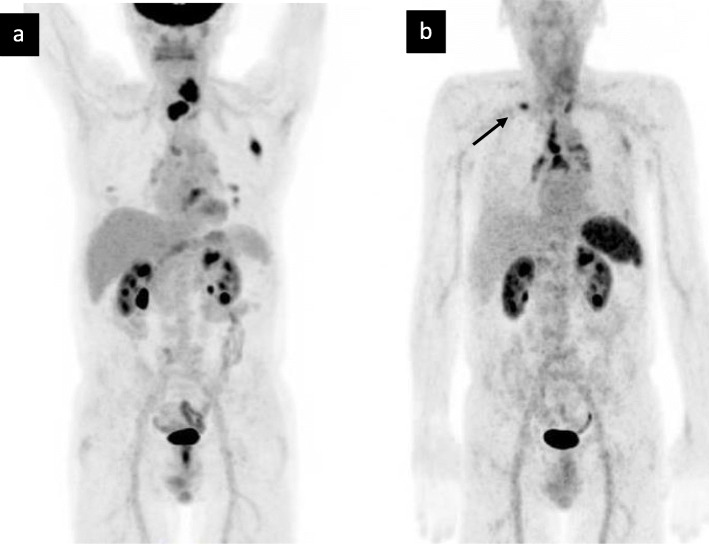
Key lesion rather peripher located to the primary tumor. Disconcordance of (a) FDG PET/CT and (b) non-invasive Pentixafor PET/CT imaging in one patient suffering from esophageal cancer. The lesion demonstrates high CXCR4 expression; local response was able to be shown in re-staging PET/CT after radiochemotherapy. Arrow indicates the lesion
